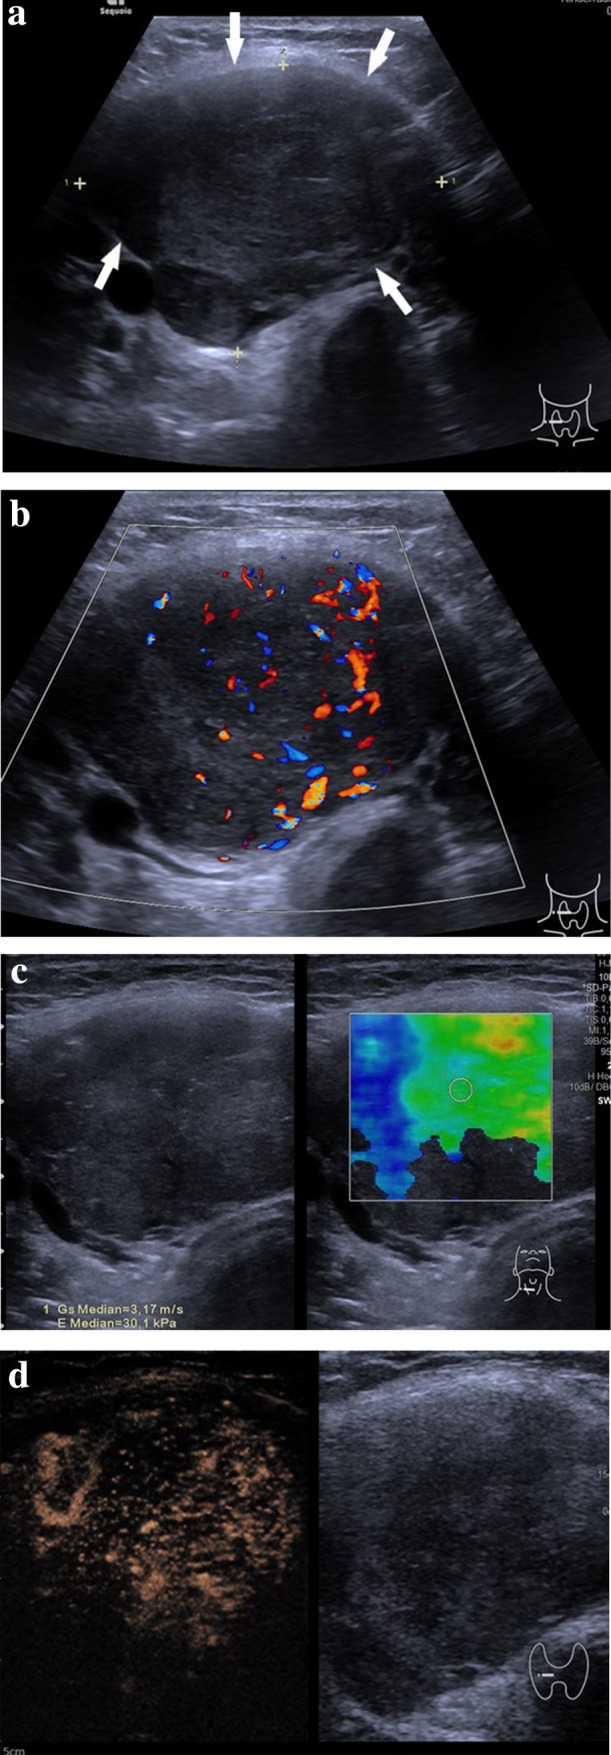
Conventional US, color Doppler US, strain elastography and CEUS images of a malignant thyroid nodule in a 15-year-old boy. a B-mode US image of an inhomogeneous slightly isoechoic to hypoechoic lesion (arrows) with ovoid-to-round shape and ill-defined margins; in the right lobe in the longitudinal section. Surgical pathology proved a medullary carcinoma. b Color Doppler US image in a transverse plane showing presence of vascularity in a malignant thyroid nodule in the right lobe. c SE image of a lesion in a transverse plane showing shear wave elastography (SWE) with increased stiffness expressed as elasticity of 30.1 kPa or high shear wave velocity of 3.17 m/s, indicating a lesion with an elevated stiffness compared to the normal thyroid parenchyma. d CEUS image in a transverse plane showing a mass with an uneven distribution of the contrast agent in the lesion indicating heterogeneous enhancement pattern
